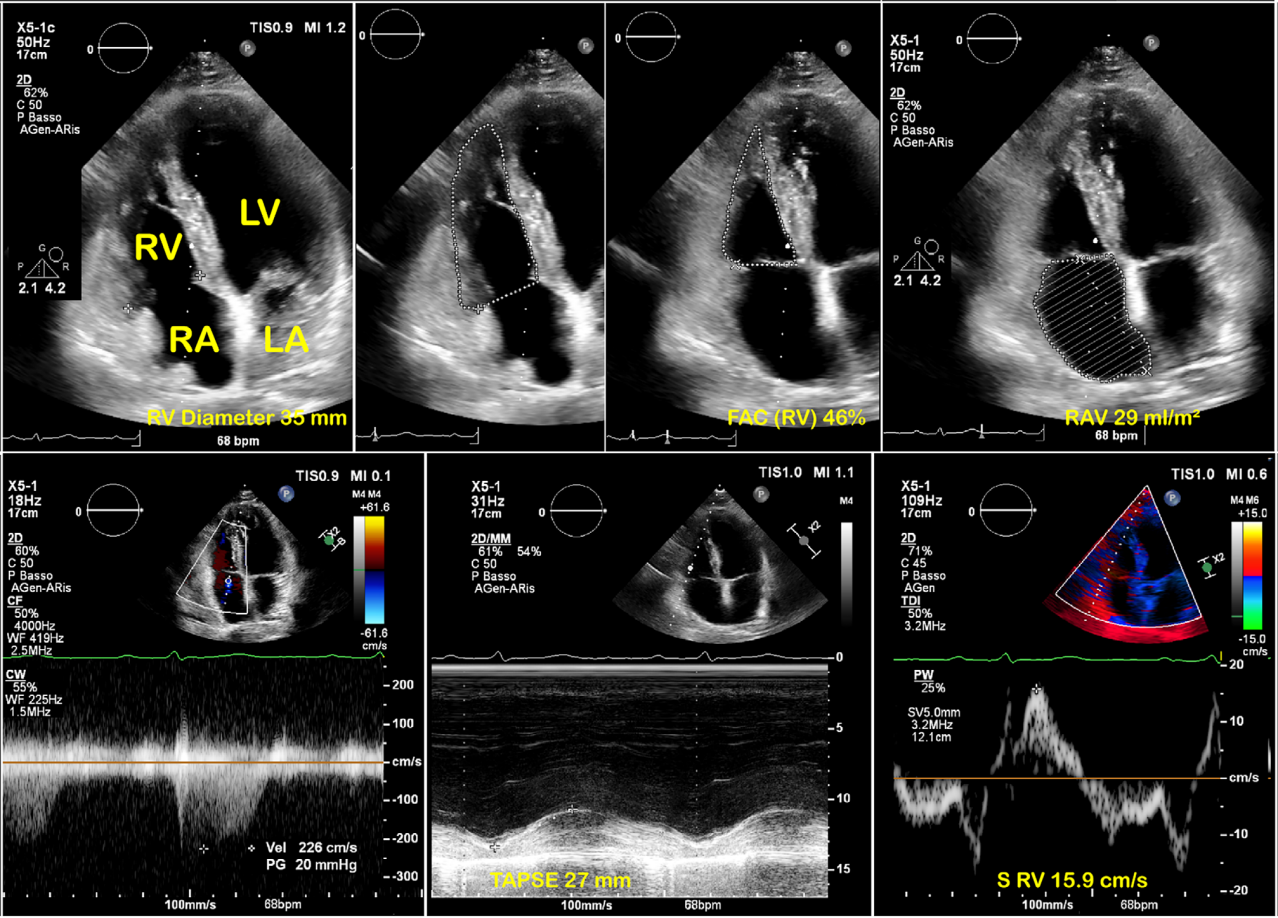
Representative two‐dimensional right‐oriented four‐chamber images to measure (from left to right) the right ventricular diameter, diastolic and systolic areas used to calculate the fractional area change (FAC), and the right atrium volume (RAV) (top panel). On the bottom panel, the continuous‐wave Doppler tricuspid flow velocity from which the tricuspid systolic pressure gradient is calculated, the tricuspid annular plane systolic excursion (TAPSE), and the systolic velocity of tricuspid annulus (S’) by tissue Doppler imaging.
